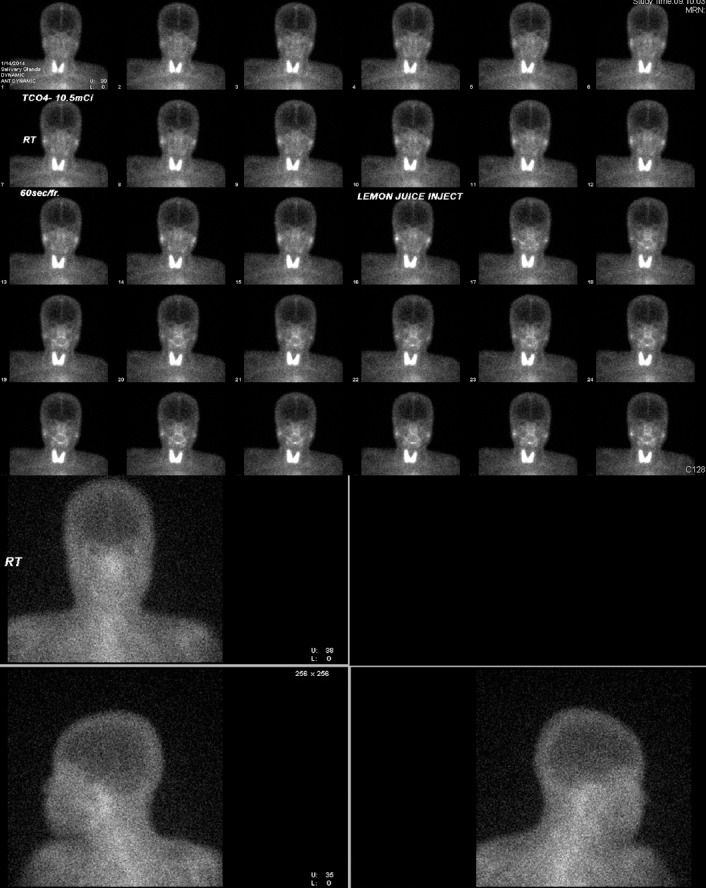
Images of a 49-year-old woman without Sjogren’s syndrome. (A) ^99m^Tc-pertechnetate scintigraphy shows good accumulation and good response for stimulation in both parotid and submandibular glands. (lemon juice at 15 min). (B) Gallium scan shows normal uptake in the bilateral parotid glands
